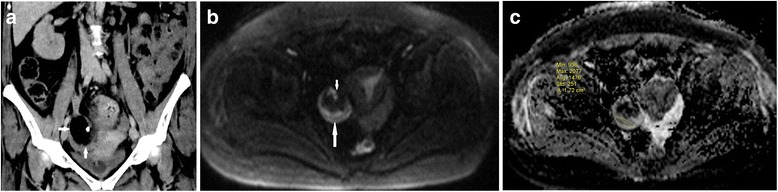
A female patient with right lower quadrant pain for three days. She had a CT scan two months ago for a different reason. At operation the right ovary was found to have slight haemorrhagic infarction on inspection and the ovary could be salvaged with partial oophorectomy. a Coronal CT scan depicts a right ovarian mass compatible with mature cystic teratoma (arrows). b b 1000 image shows an enlarged right ovary with hyperintense stroma (long arrow) containing a hypointense mass (short arrow). c ADC map reveals diffusion restriction (1.47 × 10^−3^ mm^2^/s) of the stroma of enlarged right ovary
